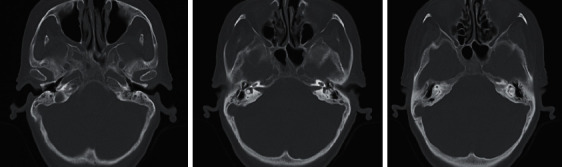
Sequential axial CT layers showing sclerotic mastoid air cells and aerated middle ears bilaterally with no evidence of effusion.
